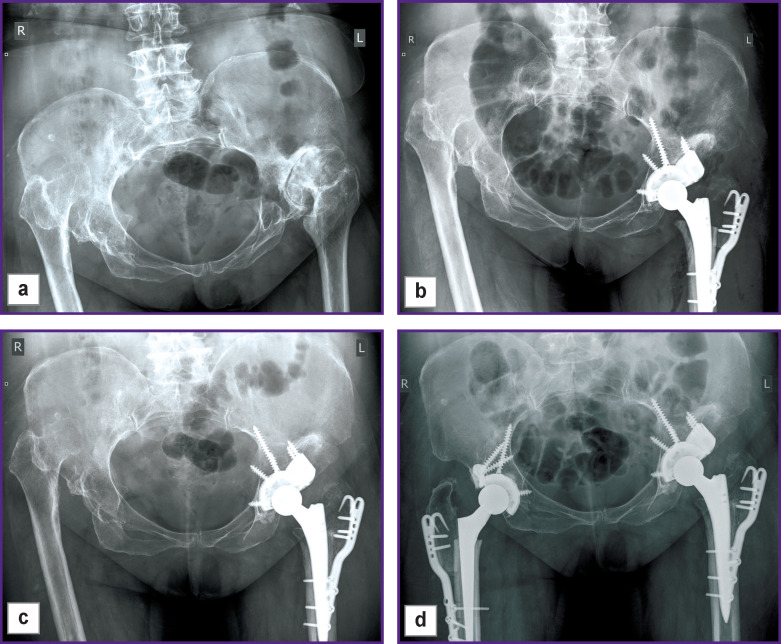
Clinical example of using the original fixator in patient S., 63 years old. Survey radiograph of the pelvis: (а) before the operation; (b) on the first day after total arthroplasty of the left hip; application of trochanteric osteotomy according to T. Paavilainen, fixation of the greater trochanter by the fork plate; (c) 6 months after the operation; radiographic signs of greater trochanter fragment consolidation with the hip are noted; (d) similar application of the method on the contralateral joint to eliminate the difference in the length of the lower limbs
